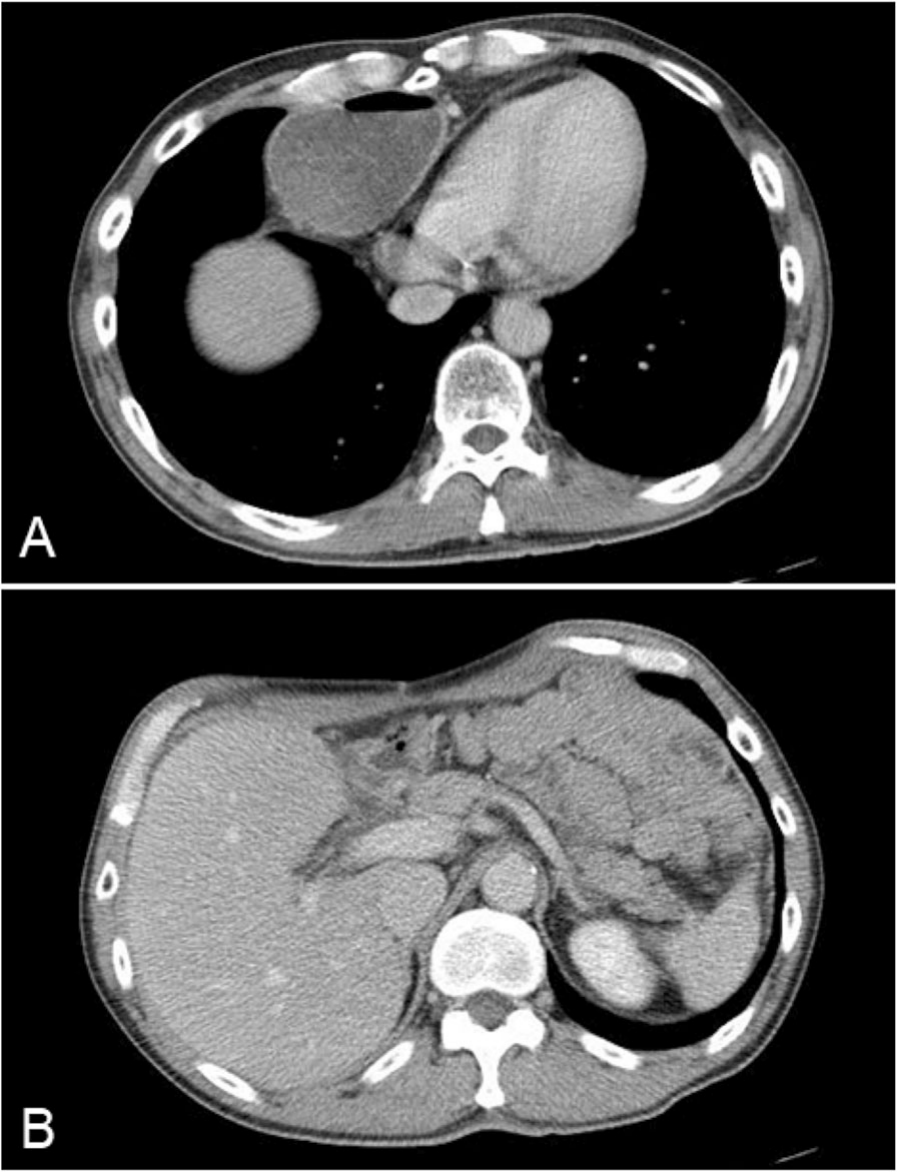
Enhanced computed tomography images. a: This image shows distension of the gastric conduit without ischemia. b: No obvious structure resembling a bezoar was observed at the pylorus ring, and there was no distension of the small intestine
